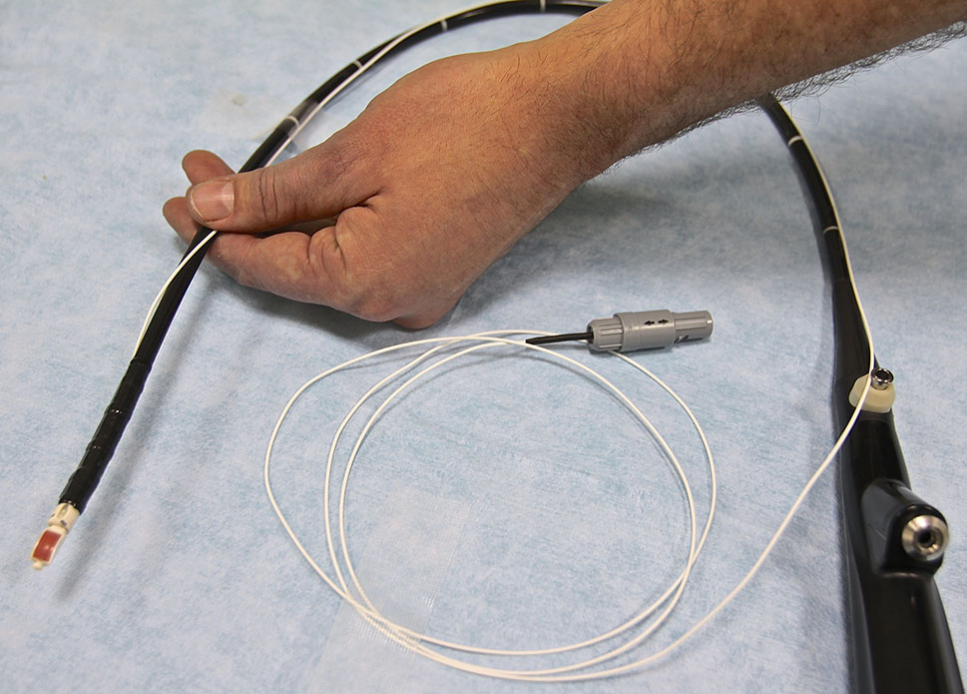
Prototype convex probe endobronchial ultrasound (CP-EBUS) bronchoscope with electromagnetic sensor integrated for position tracking
